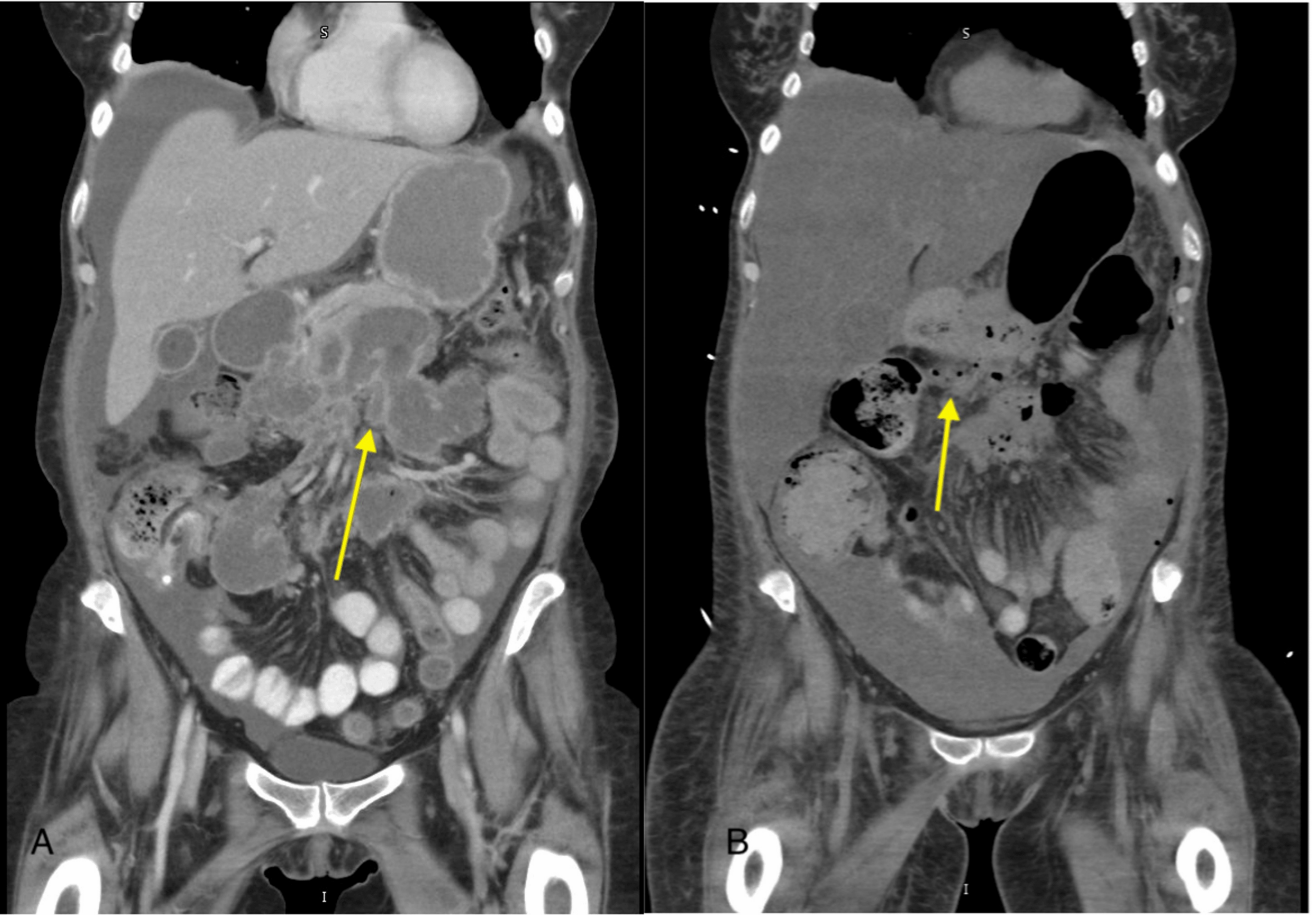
Initial CT of the abdomen and pelvis. (A) Coronal contrast-enhanced CT image of the abdomen demonstrating changes suggestive of suspected pancreatic pseudocyst (yellow arrow). (B) Coronal contrast-enhanced CT image of the abdomen demonstrating extra-luminal air (yellow arrow), indicating pneumoperitoneum likely due to perforation of a hollow viscus. CT: computed tomography
